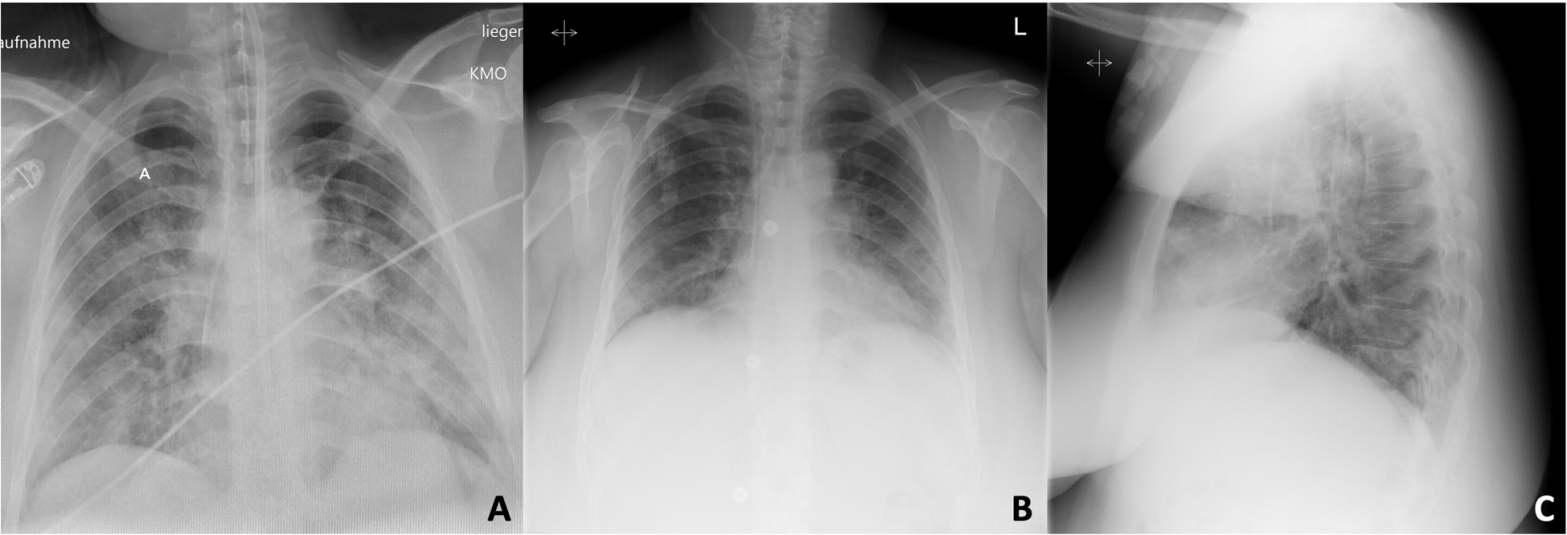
Chest radiography in a patient with ARDS due to COVID-19 before and after cesarean section: A on postoperative day 1, showing bilateral confluent opacities with basal accentuation; B on postoperative day 10, showing decreasing bilateral consolidations (ARDS: acute respiratory distress syndrome; COVID-19: coronavirus disease)
